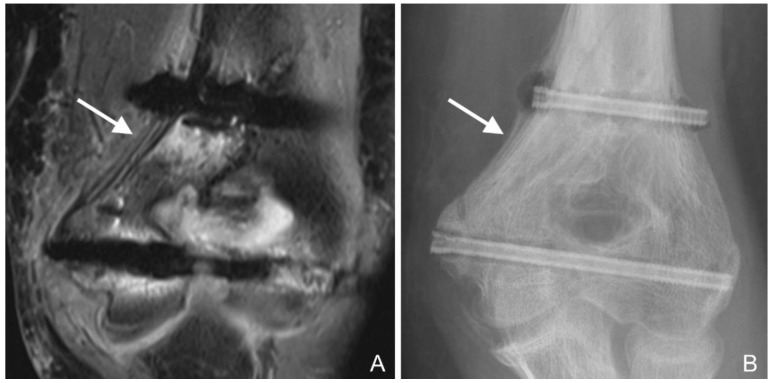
(A): PD tse fs (proton density turbo spin echo fat-saturated) coronal image of the left elbow of a 14-year-old boy with an intercondylar humeral fracture. Hypointense layers of ossified periosteal reaction alternate with hyperintense fluid (arrow). Hypointense gas locules along both screws and hyperintense bone marrow edema are also seen. (B): Corresponding radiograph. The periosteal reaction is particularly evident near the proximal screw (arrow). The gas locules along the proximal screw and at the screw head are also visible.
